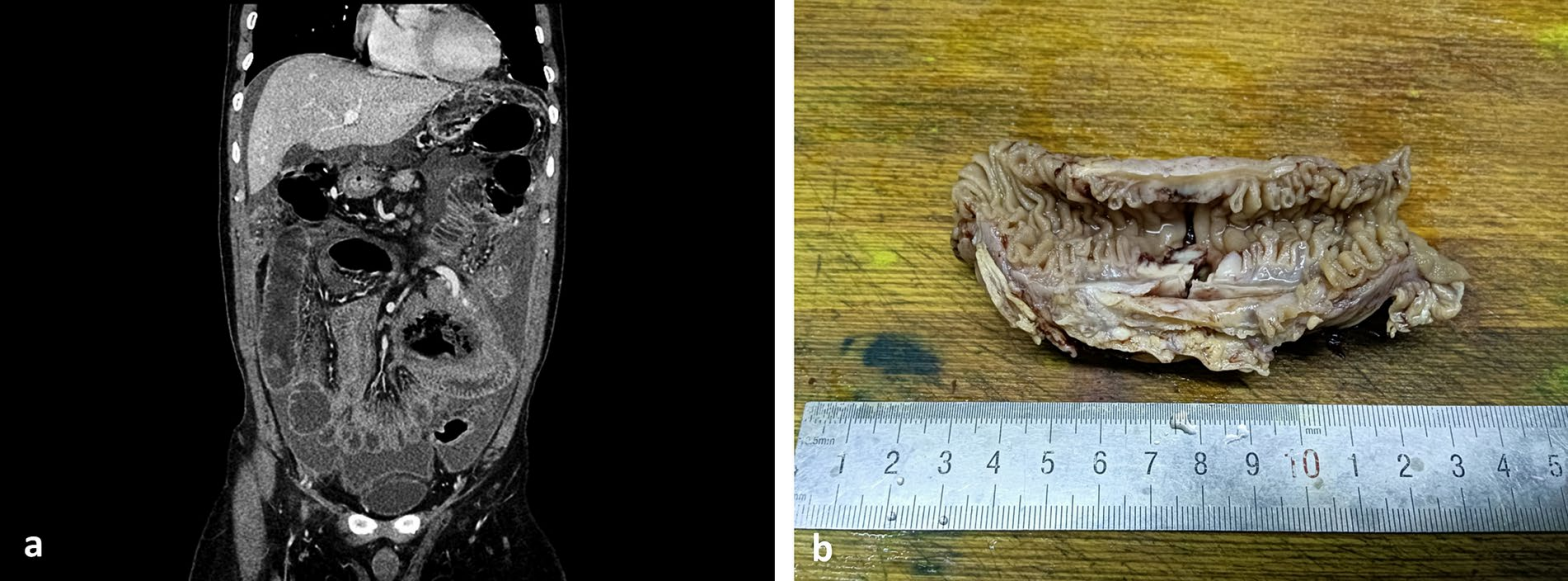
CT image and large body picture of the case. (a) CT of the upper abdomen showed peritoneal effusion with small intestinal adhesions, jejunal wall thickening, and multiple abdominal and retroperitoneal lymphadenopathies; and (b) ulcer could be seen in the membrane of the small intestine.
